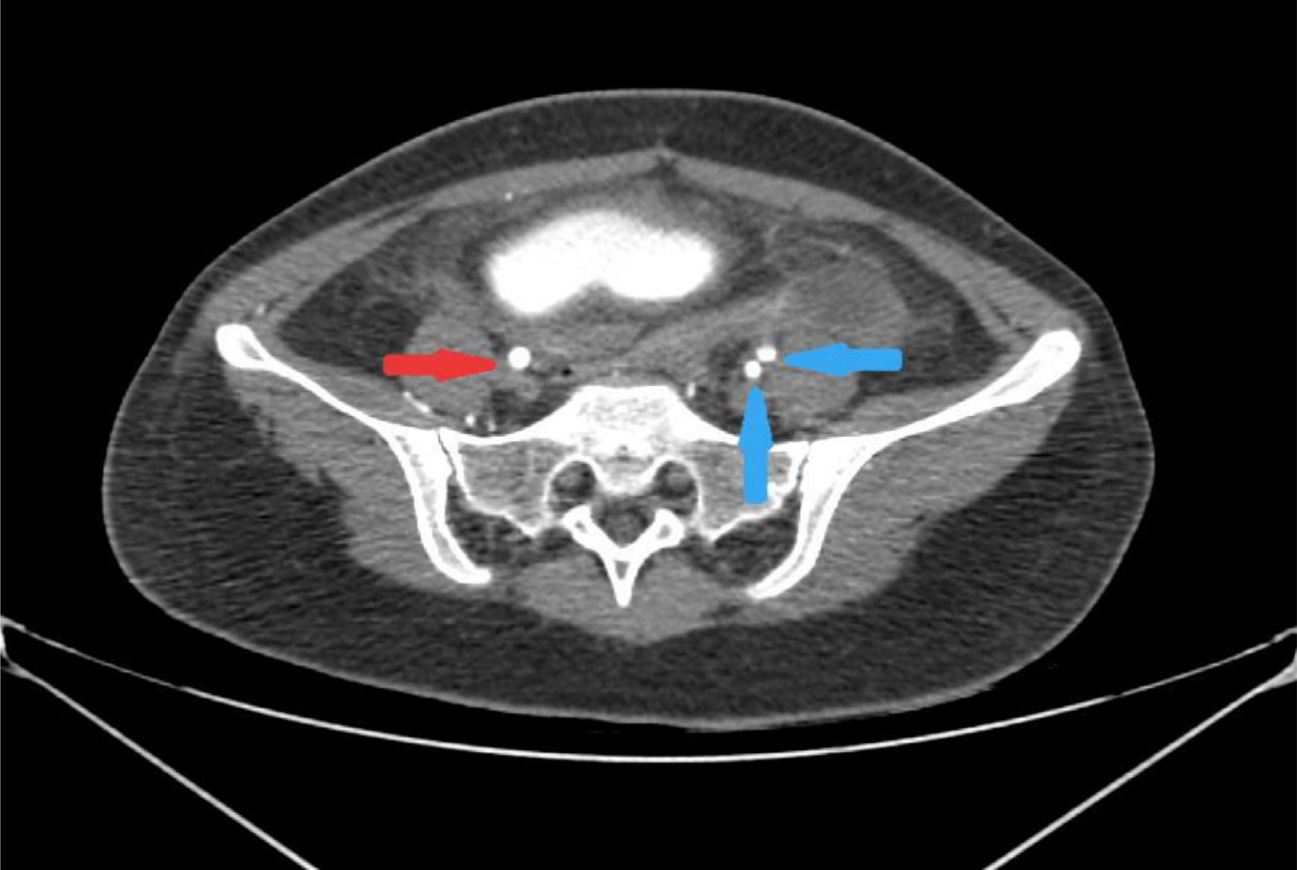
Enhanced abdominopelvic computed tomography (CT) scans; The external iliac artery on the right side was not visible (red arrow), while the left internal and external iliac arteries were visible (Blue arrow).
